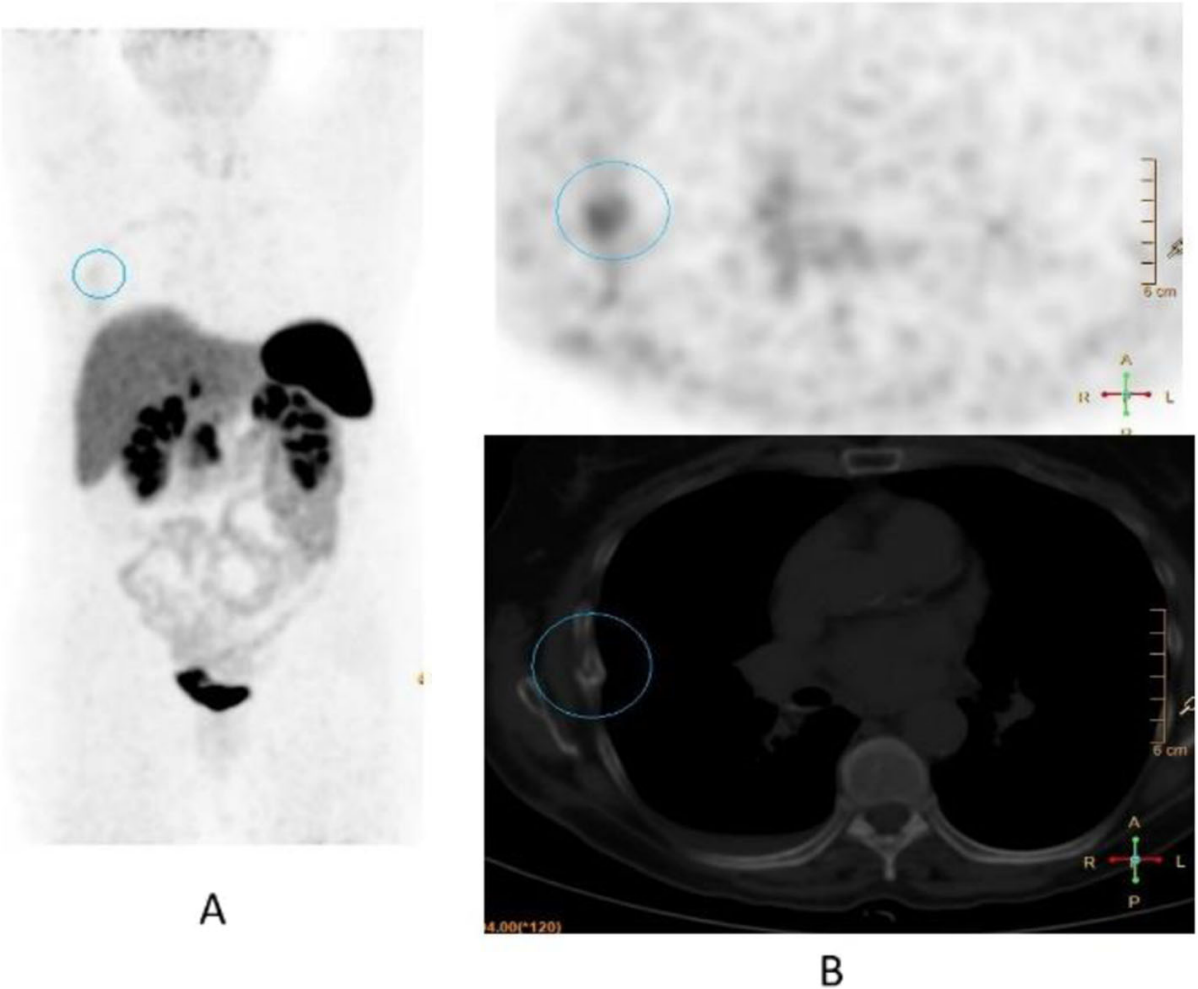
^68^Ga-DOTA peptide, restaging after surgery for lung carcinoid, specificity. *Clinical history*: 60 y.o. man; restaging after surgery for lung carcinoid. *PET/CT findings*: focal increased ^68^Ga-DOTATOC uptake in a benign rib fracture (a MIP, b PET and fused PET/CT, blue marker)
